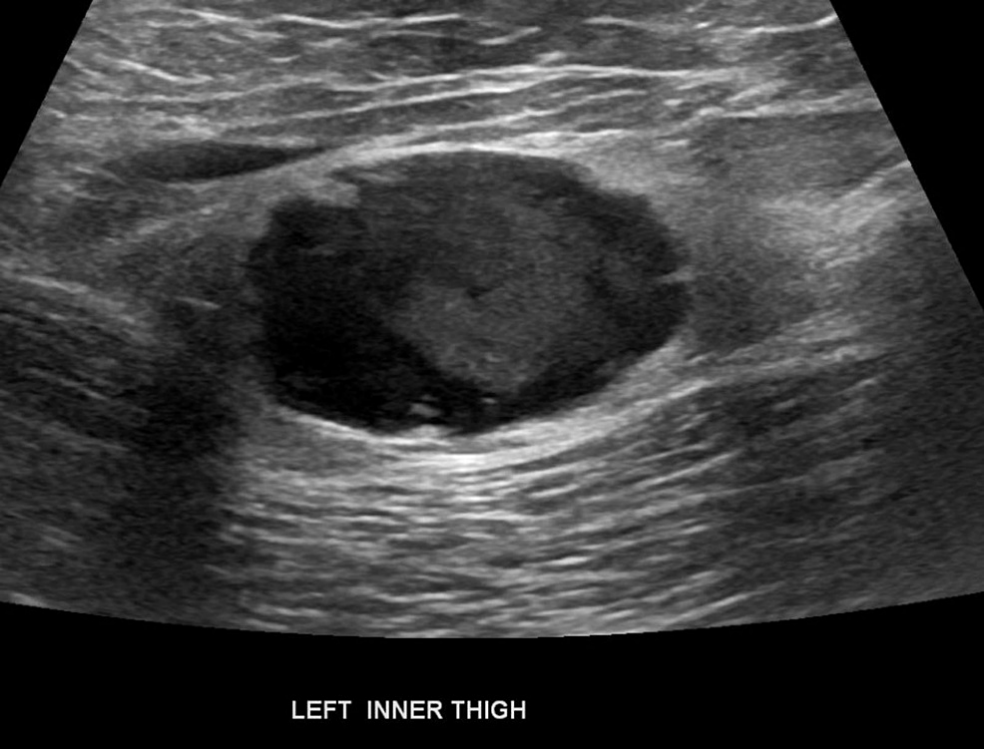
Ultrasonography showing hypoechoic mass.
